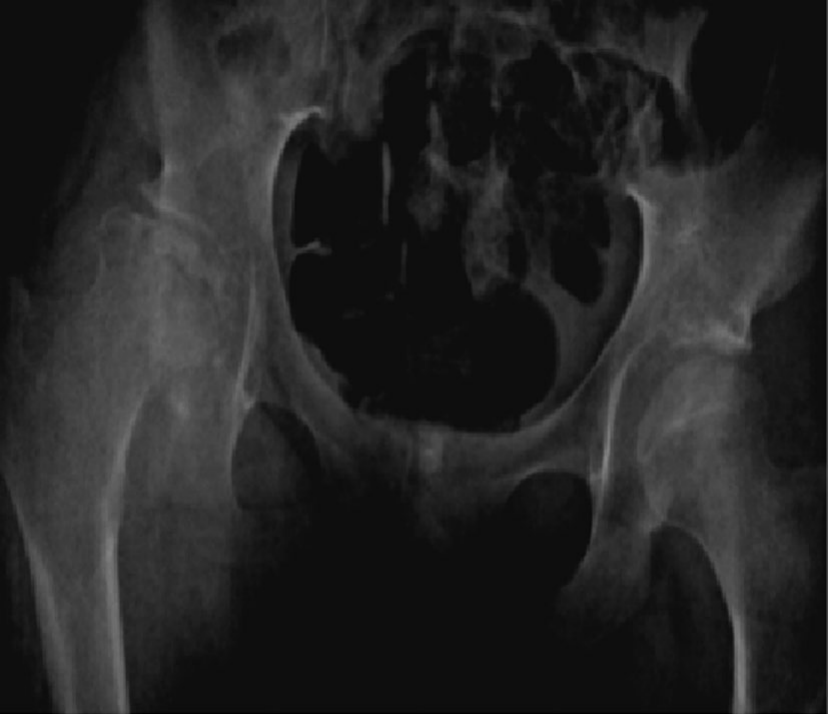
Radiograph showing elevated right hemipelvis and irregular contour of right hip-joint with lytic and sclerotic lesions in the femoral head in an SLE patient diagnosed with MDR-TB.
